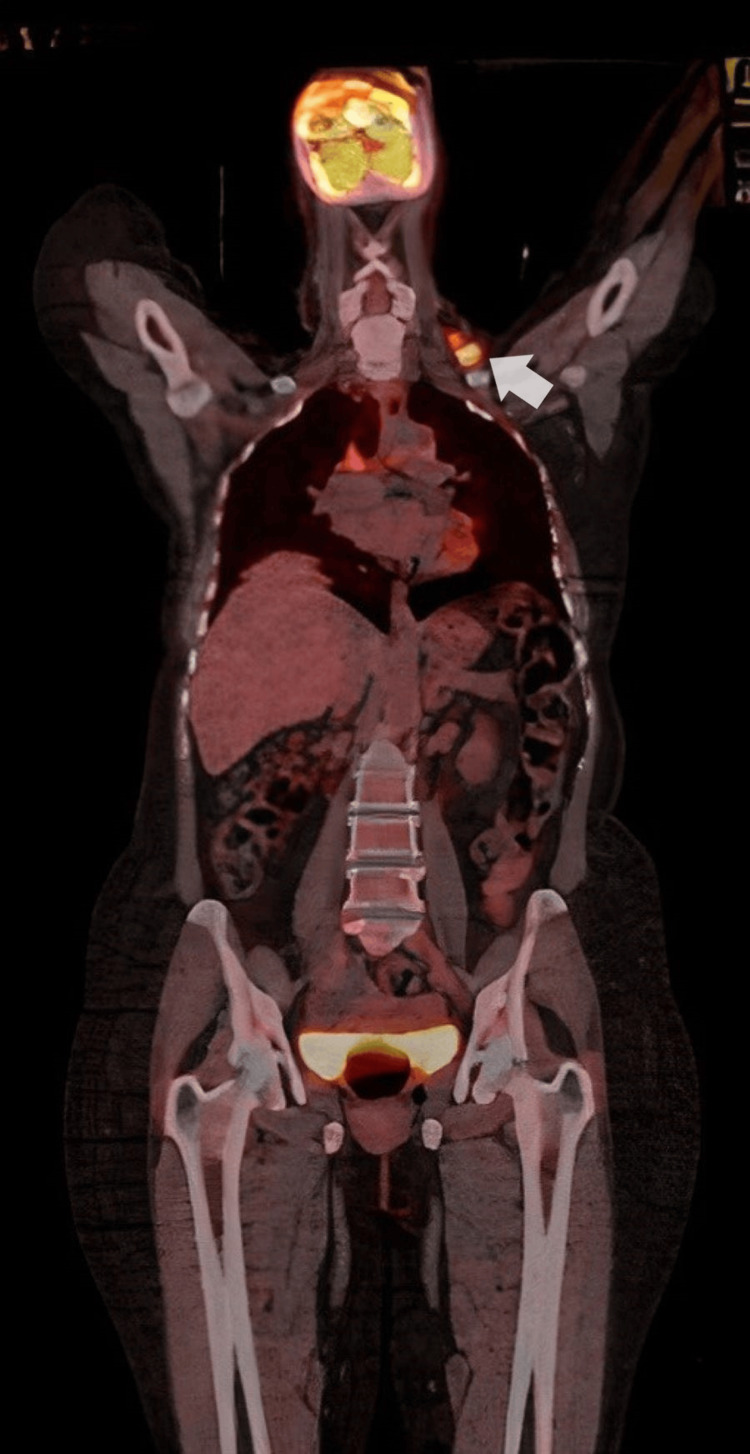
Positron emission tomography-computed tomography scan reveals a hyperintense lymph node in the supraclavicular region.
